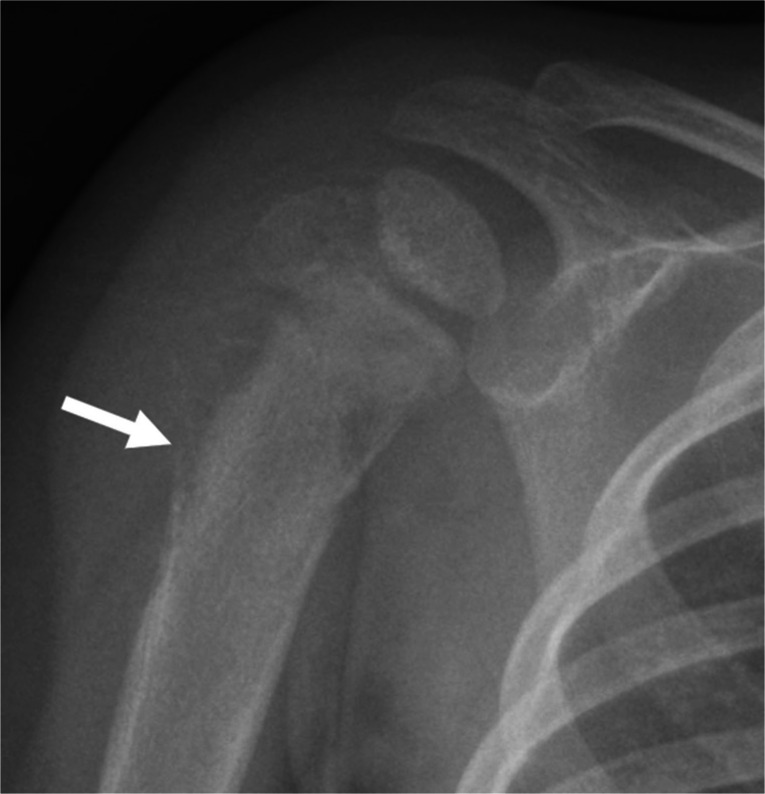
Metastatic neuroblastoma. A 1-year-old boy presented to the ED with 1 day of fussiness and decreased use of the right arm. Frontal radiograph of the right humerus shows a destructive lesion in the proximal right humerus with aggressive periosteal reaction (*arrow*). Follow-up MRI of the humerus (not shown) revealed an aggressive tumor and the patient was eventually diagnosed with metastatic neuroblastoma
